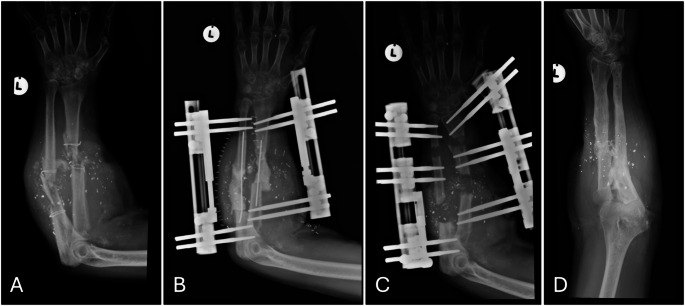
A–D. Radiographs from case 3: A Radiograph showing bullet fragments and cerclage wires in situ, and a comminuted diaphyseal fracture previously managed surgically; B Post initial debridement radiograph showing spacer in situ, held in place with intramedullary wires, and fractures stabilised with LRS fixators separately for radius and ulna bones; C Post corticotomy radiograph with proximal to distal bone transport showing early regenerate formation; D Radiograph at final follow up demonstrating full consolidation of regenerate bone
